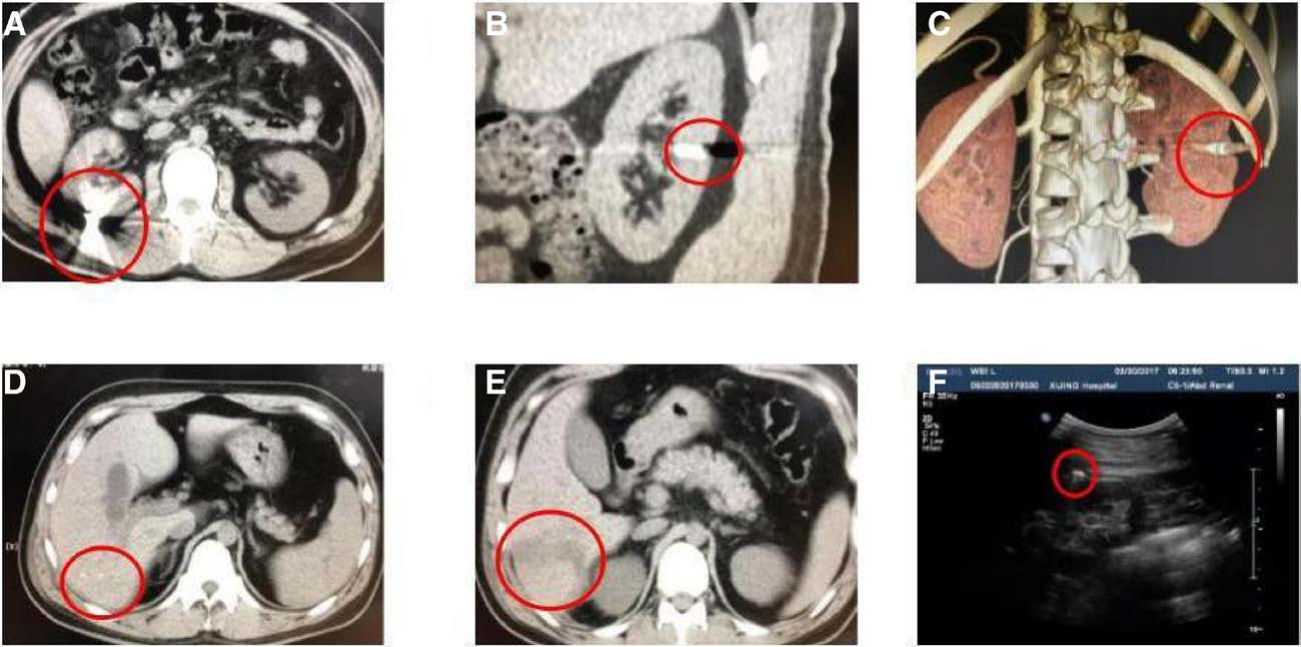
Before surgery, there was a small nodular density enhancement in the right kidney and a small calcification in the right lobe of the liver with a low-density trajectory. (A) Plain CT transverse view of right kidney. (B) Plain CT longitudinal view of right kidney. (C) Three-dimensional CT imaging of the kidney. (D) Small calcification foci in the right lobe of liver. (E) Liver low-density ballistics. (F) Right renal parenchyma ultrasonography strong echo light mass with acoustic shadow. CT = computed tomography.
